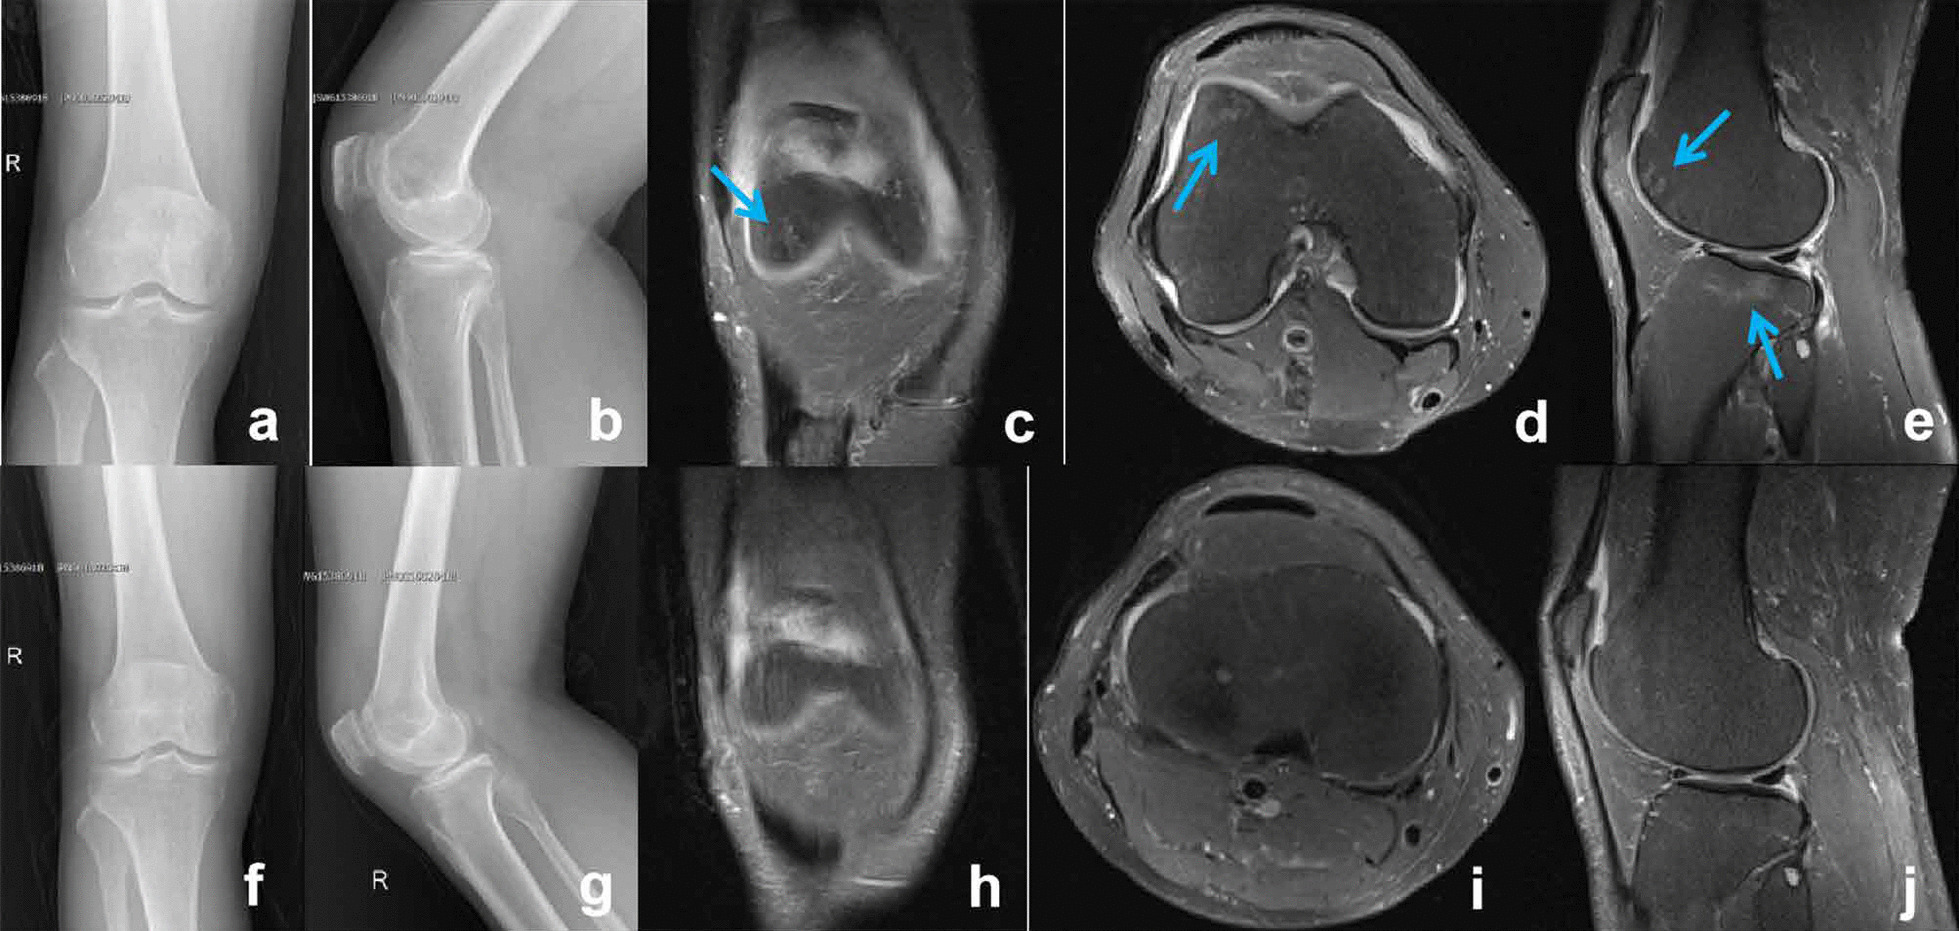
Patient 2: X-ray showed no obvious abnormality (a, b). PDWI sequence of MRI showed effusion, synovitis and bone marrow edema (blue arrow) deep to the surface of the right femoral trochlea and tibial plateau (c–e). Post-treatment X-ray images showed no obvious abnormality (f, g). Post-treatment PDWI sequence of magnetic resonance images indicates the edema of bone marrow disappeared and the effusion in articular cavity decreased significantly (h–j)
